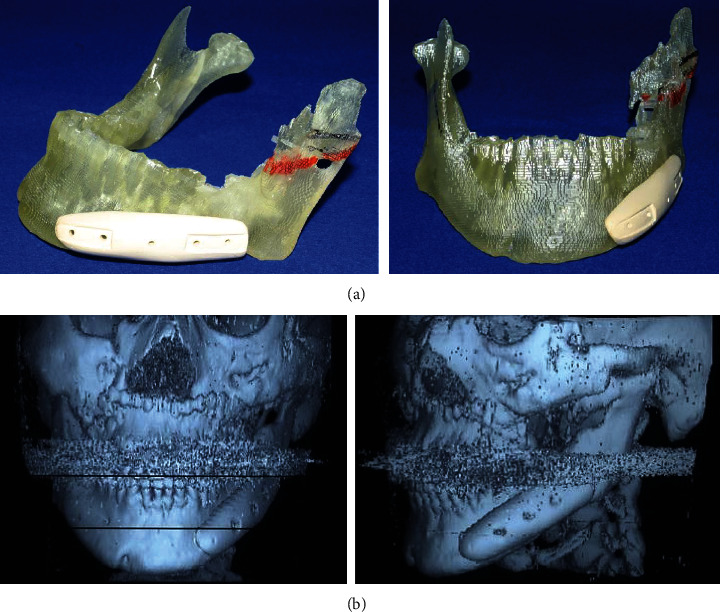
HAP-TCP blocks for onlay grafting on three-dimensional model (a) and cephalic CT images after onlay grafting of HAP-TCP block (b).
